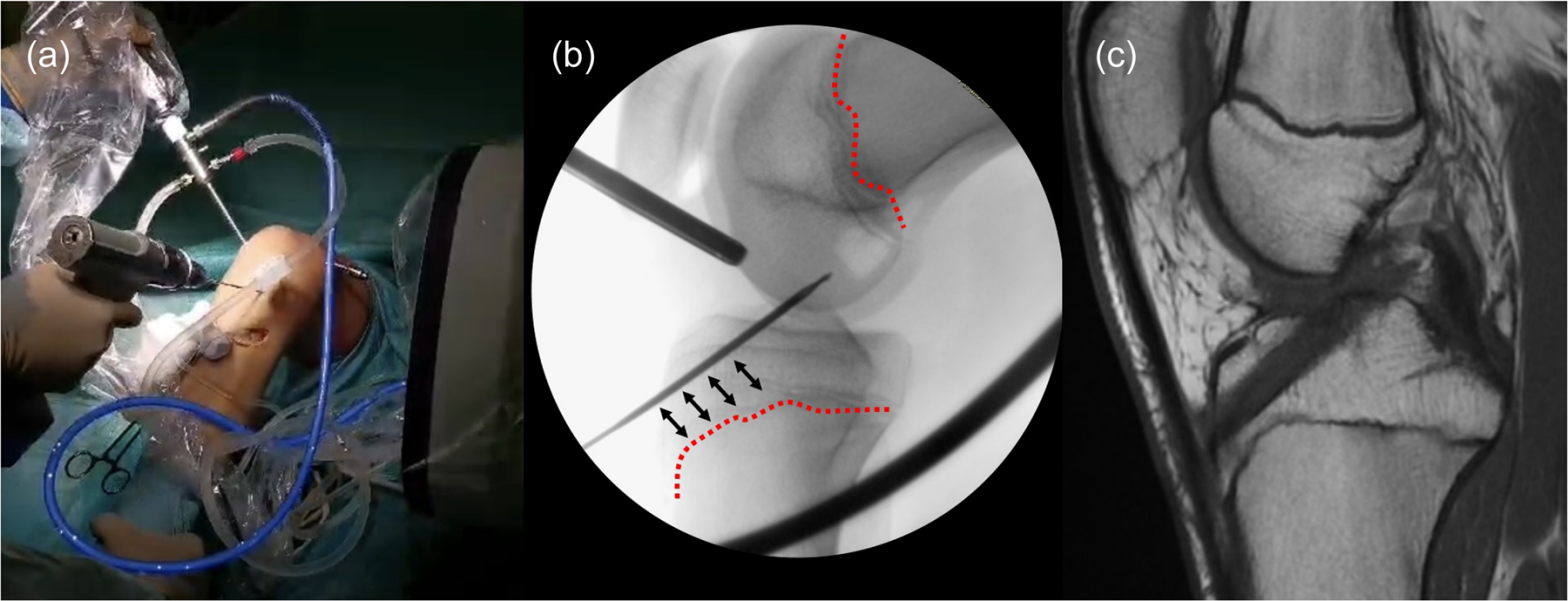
Setting for Supra‐physeal tunnel preparation, with the knee at 90° of flexion and the C‐arm perpendicular to the lower limb (a). Intra‐operative lateral x‐ray shows the placement of the k‐wire above the tibial growth plate (red dotted line) and within the proximal tibia epiphysis (b). Post‐operative MRI shows no conflict of the tibial tunnel with the tibial growth plate (c). MRI, magnetic resonance imaging.
